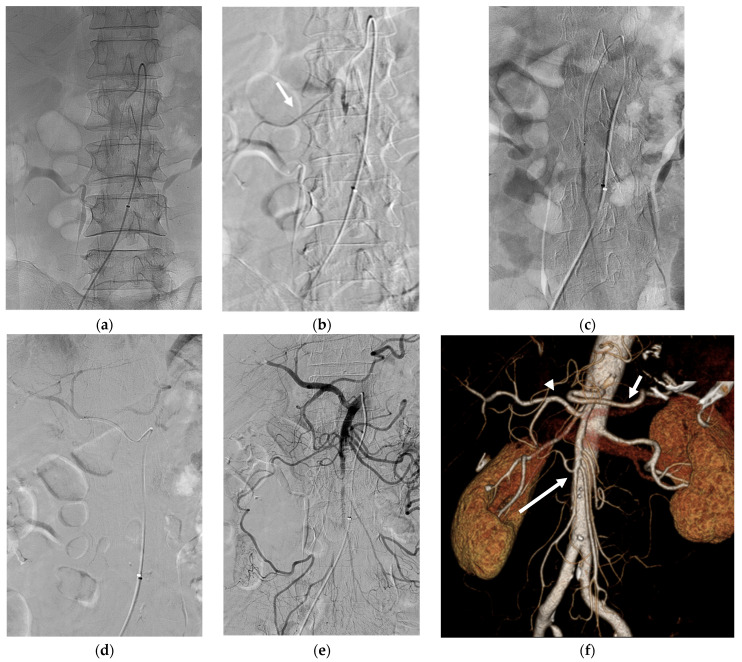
(a) The 1.9Fr microcatheter was advanced in front of the SMA occlusion site and 0.5 mg of rtPA was injected for 10 min. (b) After the injection, angiography revealed partial recanalization of right colic flow (arrow). (c) The microcatheter was then advanced into the middle and distal SMA and 0.5 mg of rtPA was injected for 10 min. (d) The CHA was also selectively catheterized using the microcatheter and 0.5 mg of rtPA was injected for 10 min. (e) Final angiography revealed near total recanalization of SMA and its branches. (f) After 2 days, follow-up three-dimensional volume-rendered image of abdominal CT angiography revealed successful recanalization of the SMA (long arrow), the CHA (arrowhead), and the splenic artery (short arrow).
